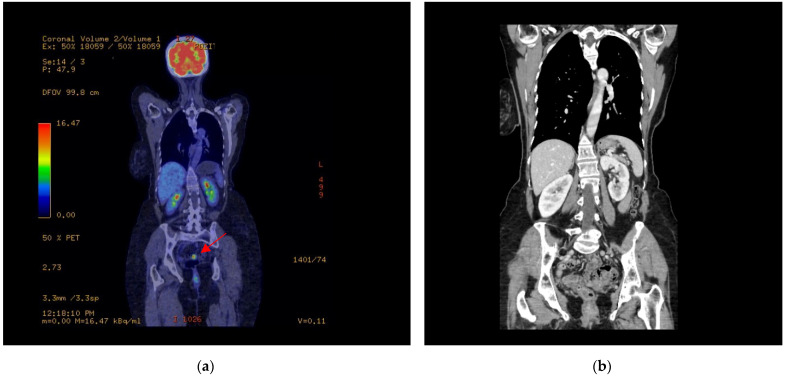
(a) Coronal fused PET/CT image demonstrating metabolic activity throughout the body. Physiological uptake is observed in the brain, kidneys, and bladder. A focal area of increased FDG uptake is identified in the lower abdomen, corresponding to the sigmoid colon (arrow), consistent with a metabolically active lesion; (b) Coronal contrast-enhanced CT scan of the thoracoabdominal region. The sigmoid colon is visible in the lower abdomen, with no obvious signs of obstruction or perforation. No lymphadenopathy or distant organ lesions are noted.
